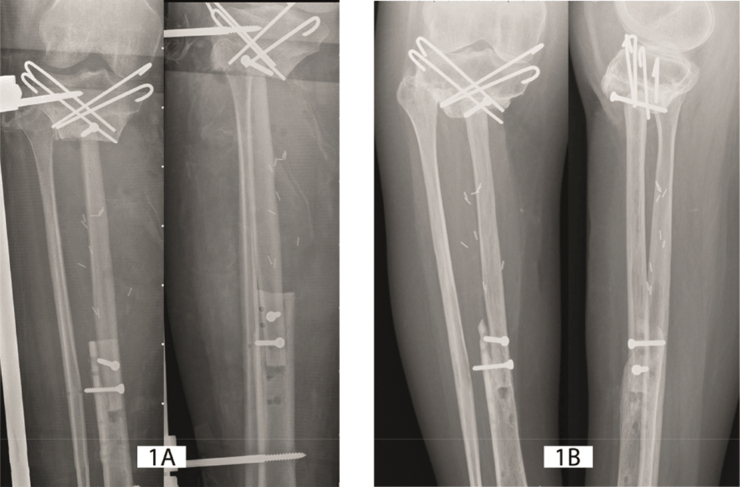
A. Early postoperative AP and lateral X-rays of a young female patient with tibial diaphyseal reconstruction following resection of a malignant bone tumor. This case was complicated by early postoperative arterial thrombosis, rendering the flap avascular. B. X-rays taken during the 26^th^ postoperative month reveal slight atrophy with diminished bone density at the flap, despite a successful union of the flap host interfaces.
